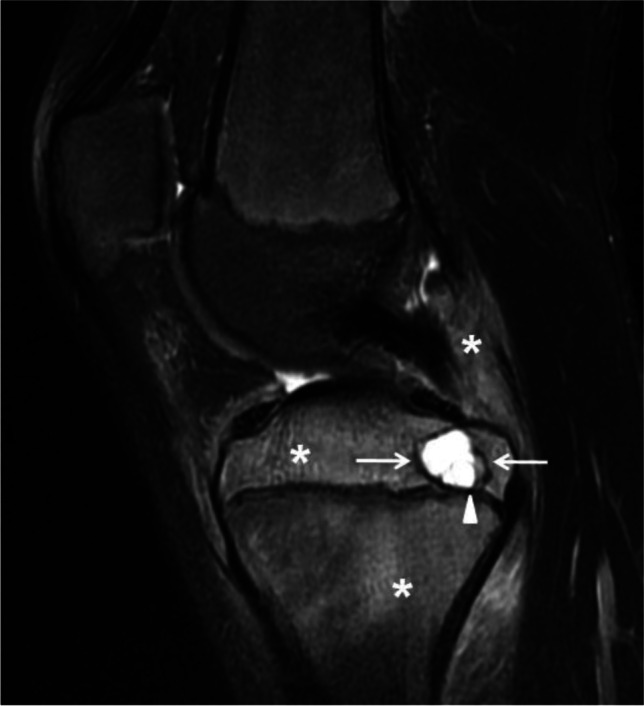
Chondroblastoma containing aneurysmal bone cyst (ABC)-like changes in a 13-year-old boy. A sagittal fat-suppressed T2-weighted magnetic resonance image of the knee shows a well-defined, lobulated lesion involving the posterior aspect of the proximal tibial epiphysis (*arrows*) corresponding to the chondroblastoma. The lesion is cystic and multiseptated containing a fluid-fluid level (*arrowhead*). Note the extensive, surrounding bone marrow and soft-tissue edema (*asterisks*) characteristic of chondroblastomas. After resection, the diagnosis of chondroblastoma with ABC-like changes was confirmed
